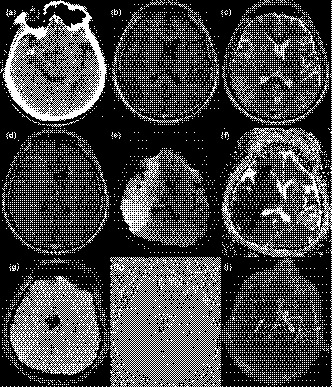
Male, 64 years. (a) CT shows high‐density performance of the right middle cerebral artery on admission at 8 hr from onset of symptoms(arrow). (b–d) T1 weighted imaging(T1WI), T2 weighted imaging(T2WI) and Fluid‐attenuated inversion recovery (FLAIR)imaging shows the right cerebral hemisphere with abnormal signal after admission, about 24 hr since onset of symptoms. (e and f) Diffusion‐weighted imaging (DWI) shows the lesions with high signal, corresponding to apparent diffusion coefficient (ADC) undervalued after admission, about 24 hr since onset of symptoms. (g and h) Susceptibility‐weighted imaging (SWI) shows hemorrhage of the thalamus after admission, about 24 hr since onset of symptoms. (i) Magnetic resonance angiography (MRA) shows occlusions involving the right middle cerebral artery and internal carotid artery (arrow), and double focal stenosis of the anterior cerebral artery, posterior cerebral artery, and left middle cerebral artery focal
